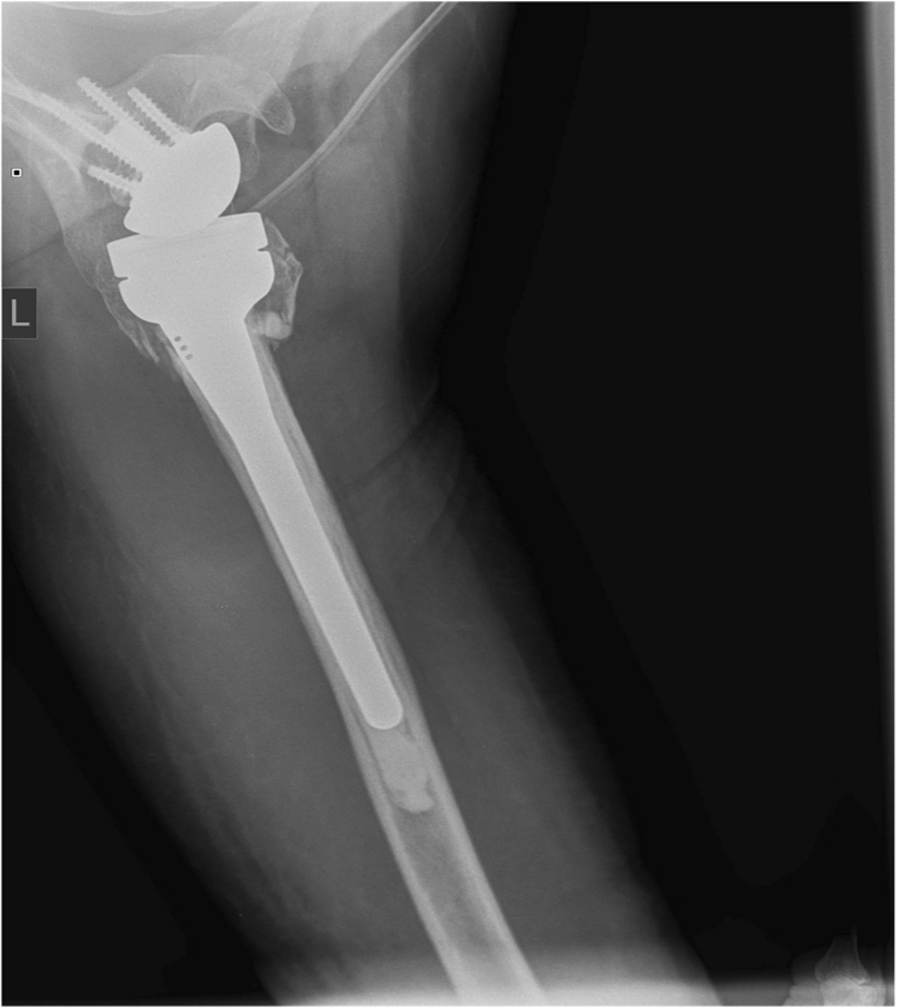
X-ray after implantation of reverse shoulder arthroplasty (axial-view).
